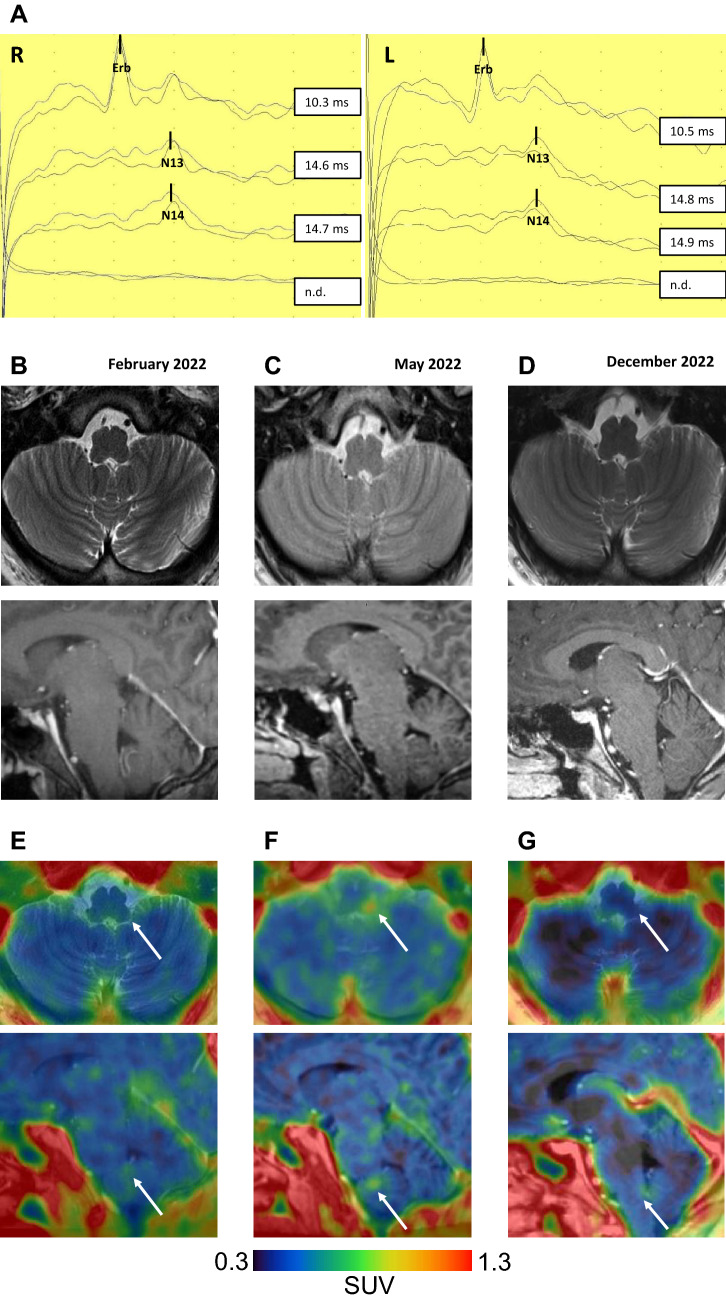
Neurophysiology and -imaging of the patient. A Somatosensory evoked potentials of the right (R) and left (L) median nerve and corresponding measured latencies. Stimulus responses were acquired at Erb’s point (Erb), C7 (N13), C2 (N14) and cortical (N20). Horizontal interval between dots: 50 ms. Vertical interval between dots: 2 µV. n.d. = not detectable. B–D Axial T2 and sagittal contrast enhanced T1 MRI-sequences of the brain with TSPO-PET scan overlays. E–G Images were acquired at baseline in February after prednisone-pulse therapy (left column), clinical deterioration in May (middle column) and after cyclophosphamide therapy in December 2022 (right column). Tracer enhancement (white arrows) was measured by standardized uptake value (SUV) in a tracer-dosage- and bodyweight-dependent manner. The SUV are absolute values without normalization to a reference region. All TSPO-images were displayed with a uniform SUV-range of 0.3–1.3 to allow visual comparability. Faint focal tracer uptake was detected visually mainly in sagittal view within the dorsal medulla oblongata at baseline (SUV: 0.44; E). Clinical deterioration correlated with progressive enhanced microglia activation within the dorsal pons and medulla oblongata (SUV: 0.71; F). After cyclophosphamide therapy, no pathological tracer uptake was measured anymore (SUV: 0.41; G)
